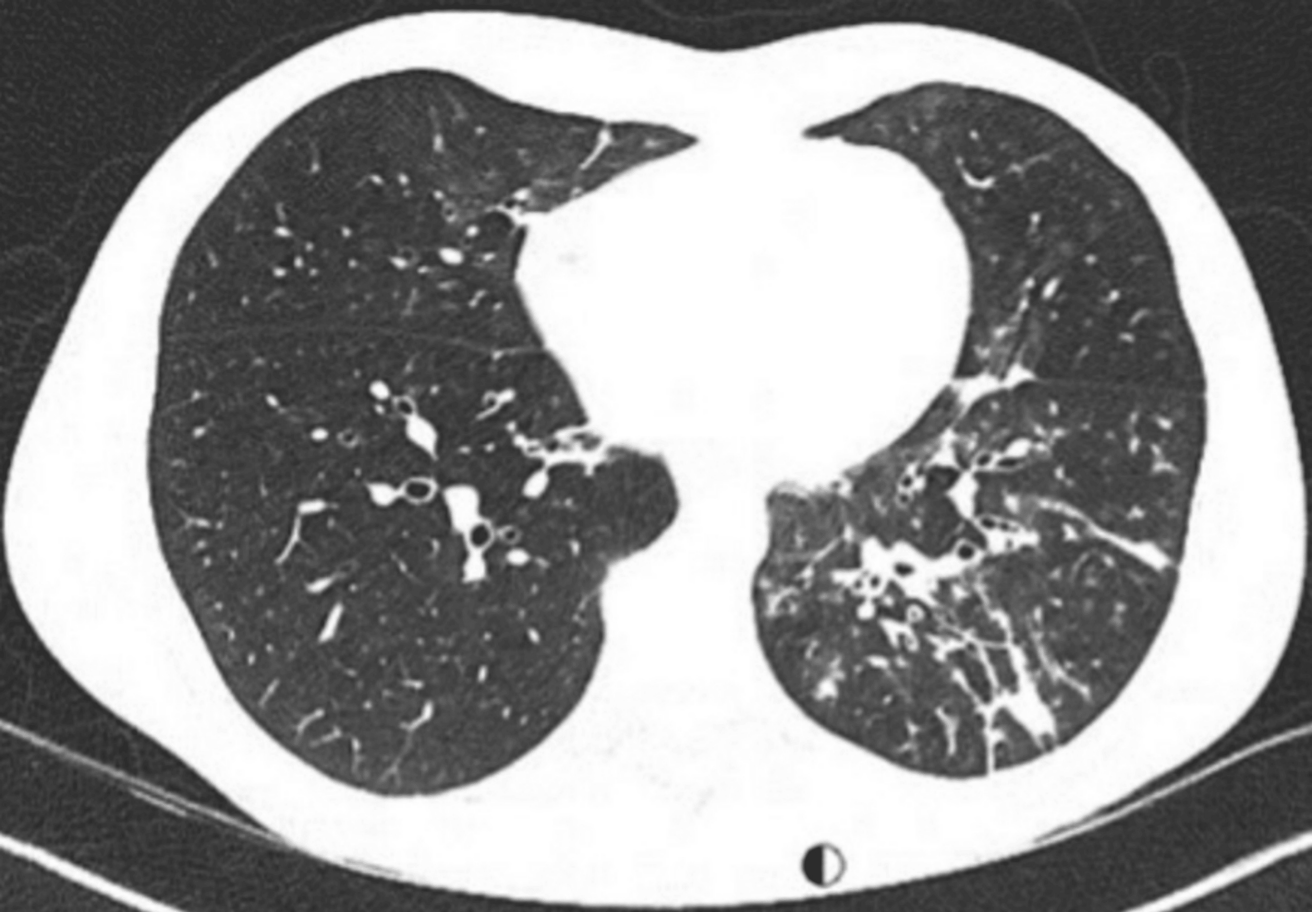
CT pulmonary angiogram showing left lower lobe and to a lesser degree right lower lobe consolidation with airway mucus plugging, diffuse nodularity, and a mild degree of ground-glass change within both lower lobes, worse in the left.
